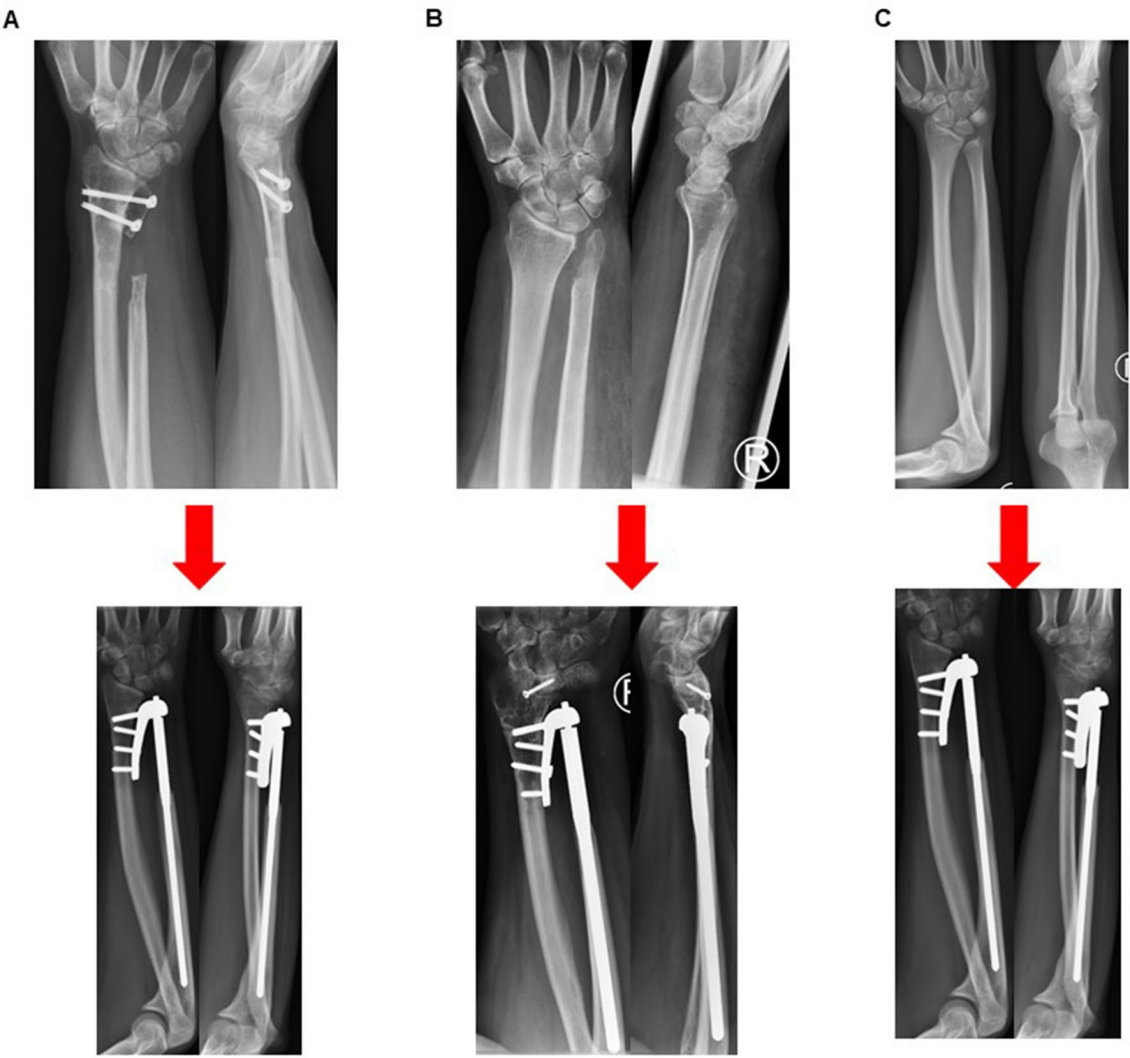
Radiograph of a wrist after Sauvé-Kapandji procedure fusing the ulna head to the distal radius and resecting a part of the ulna shaft to still enable rotation in the forearm, on the bottom pictures after implantation of an Aptis DRUJ prosthesis. Radiograph of a wrist after Bowers procedure with hemiresection of the ulna head and interposition arthroplasty on the bottom pictures after implantation of an Aptis DRUJ prosthesis. Radiograph of an acquired or congenital deformity of the proximal radioulna joint causing DRUJ pain on the bottom pictures after implantation of an Aptis DRUJ prosthesis
